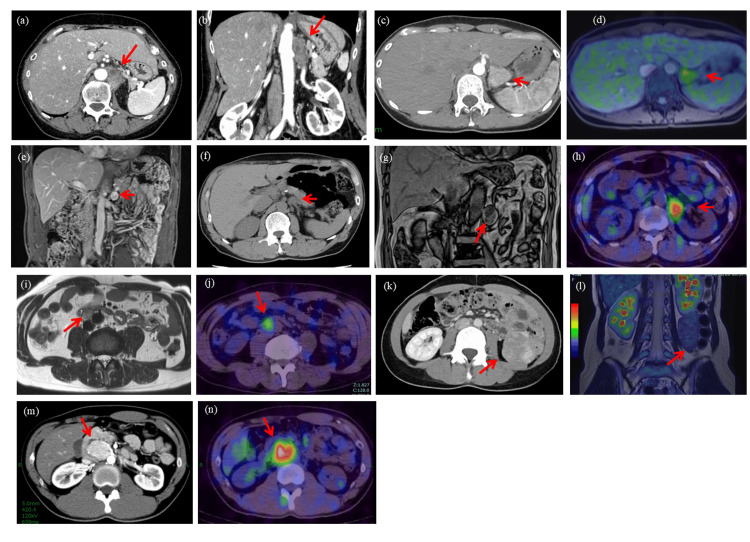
Preoperative imaging findings of all tumors (red arrows) (a, b) Case 1: preoperative axial and coronal CT images showing a tumor surrounding the left renal vessels on the left side of the aorta; (c, d) Case 2: preoperative axial CT and FDG-PET images; (e, f) Case 3: preoperative coronal MRI and axial CT images; (g, h) Case 4: preoperative coronal MRI and axial I123-MIBG scintigraphy images; (i, j) Case 5: preoperative axial MRI and I123-MIBG scintigraphy images; (k, l) Case 6: preoperative axial CT and coronal FDG-PET images; (m, n) Case 7: preoperative axial CT and I123-MIBG scintigraphy images I123-MIBG: iodine-123 metaiodobenzylguanidine
